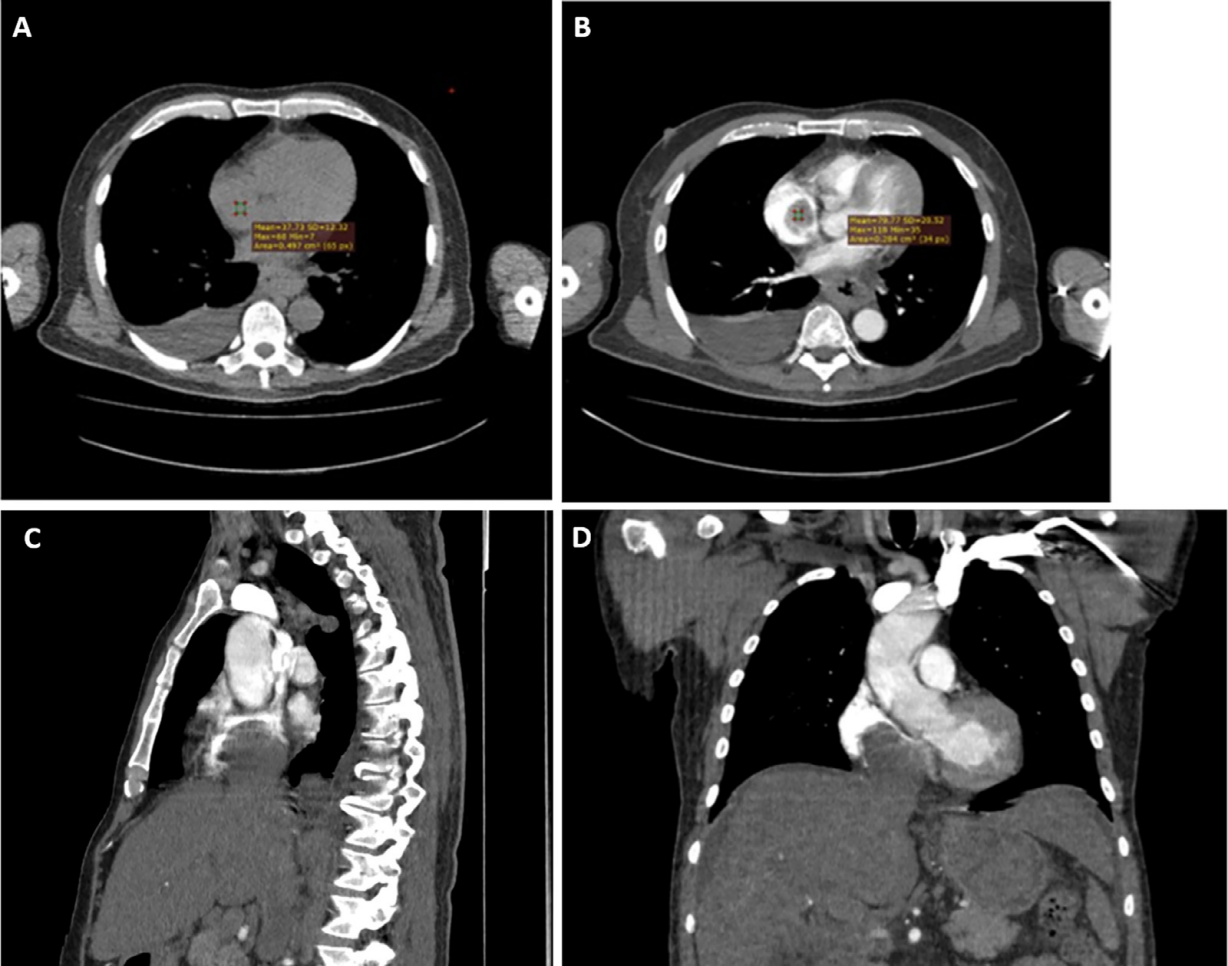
(A) Computed tomography pulmonary angiography before contrast enhancement showed that the mass extends from inferior vena cava to the right atrium, (B) Computed tomography pulmonary angiography after contrast enhancement revealed that this mass enhances with contrast agent, indicating that this mass may be a tumor thrombus, (C) Sagittal view showing the tumor thrombus extending from inferior vena cava to the right atrium, (D) Coronal view showing the tumor thrombus extending from inferior vena cava to the right atrium.
